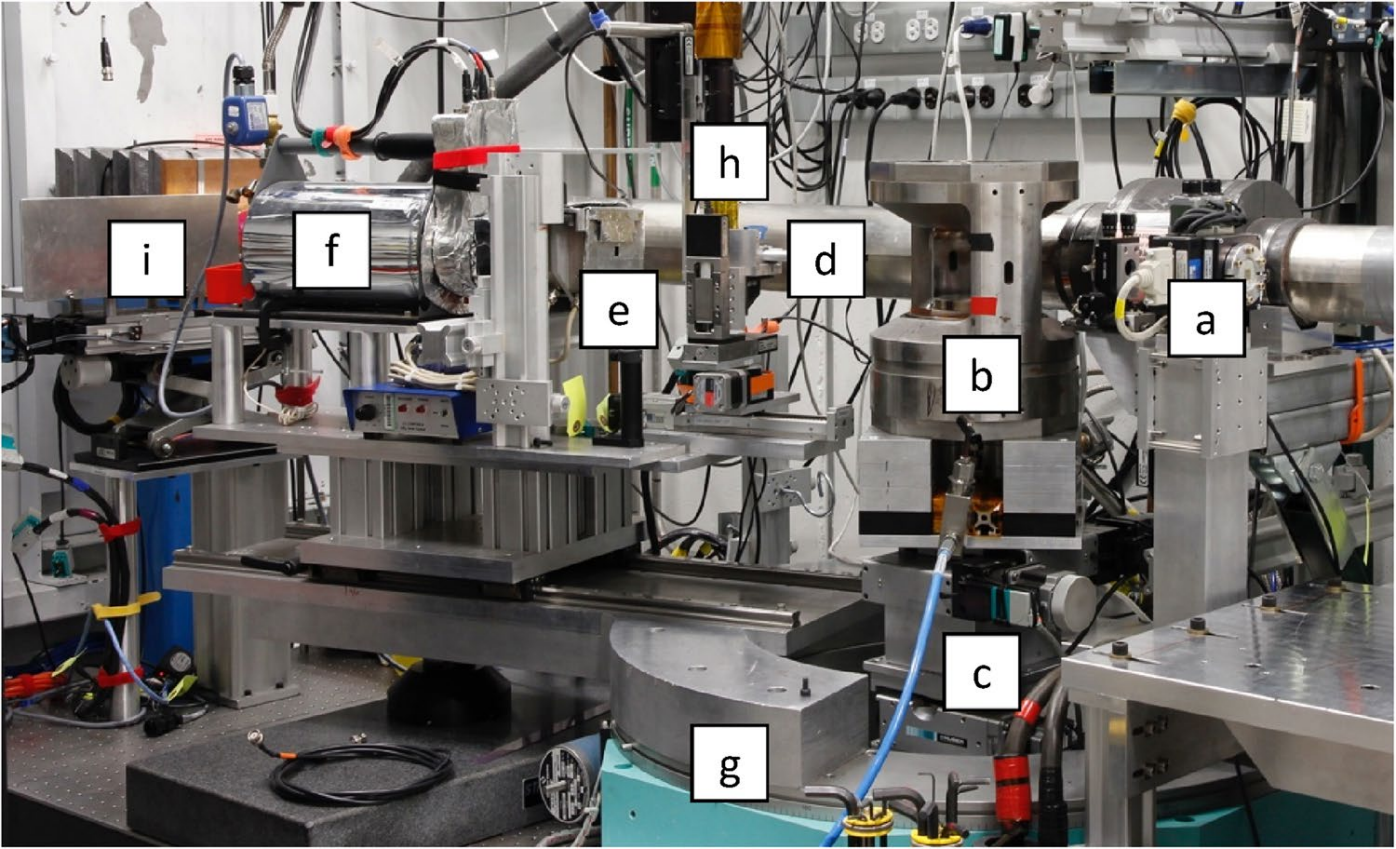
PE press setup at 16-BM-B: a collimating slits; b PE press; c positional scanning stages; d EDXD collimating tip; e EDXD detector-side collimating slits; f EDXD detector; g EDXD detector 2θ motion goniometer; h high resolution radiography system; i high speed radiography system
